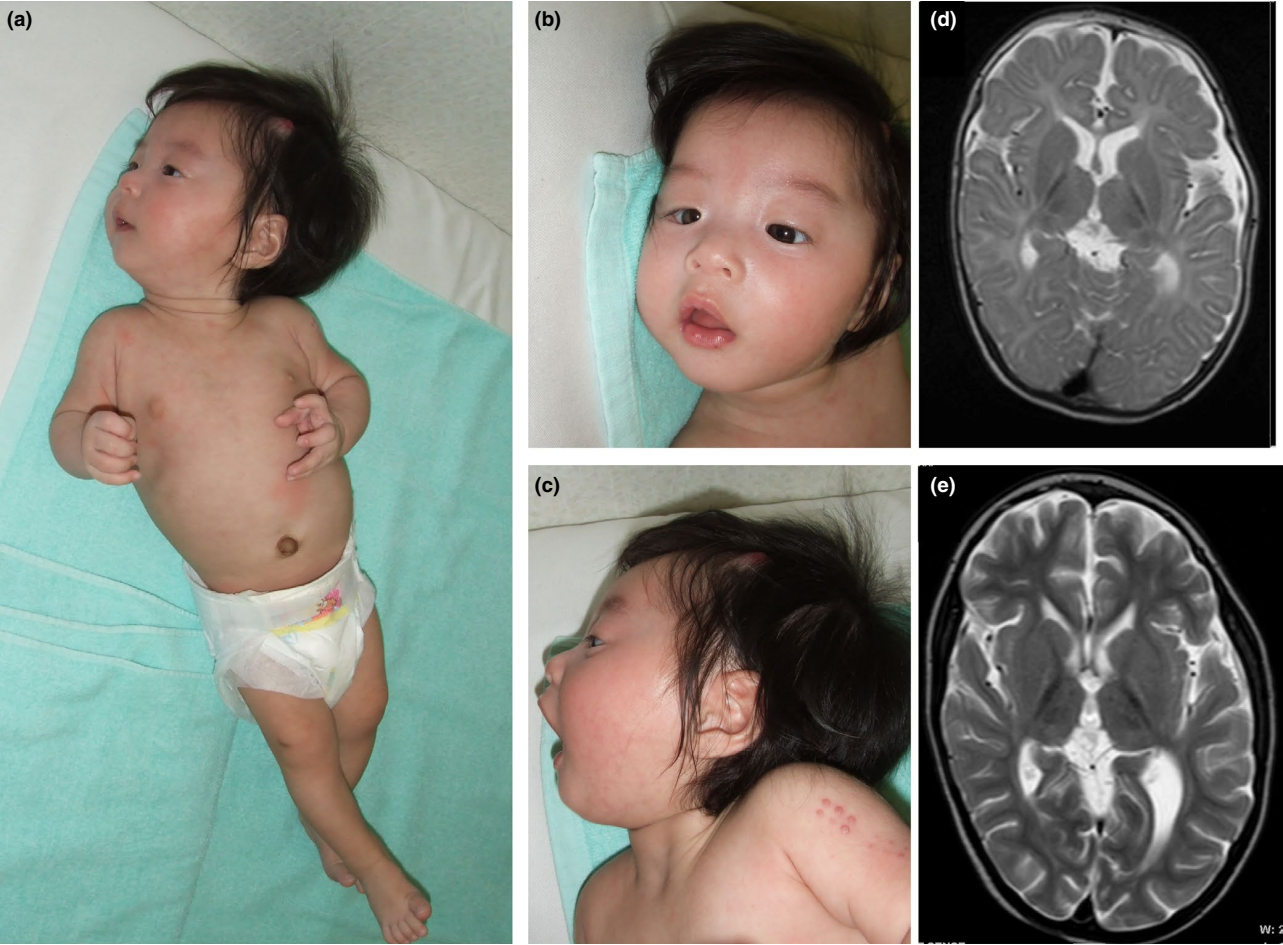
Phenotype of the patient at the age of 6 months (a‐c) and his MRI findings (d,e)
